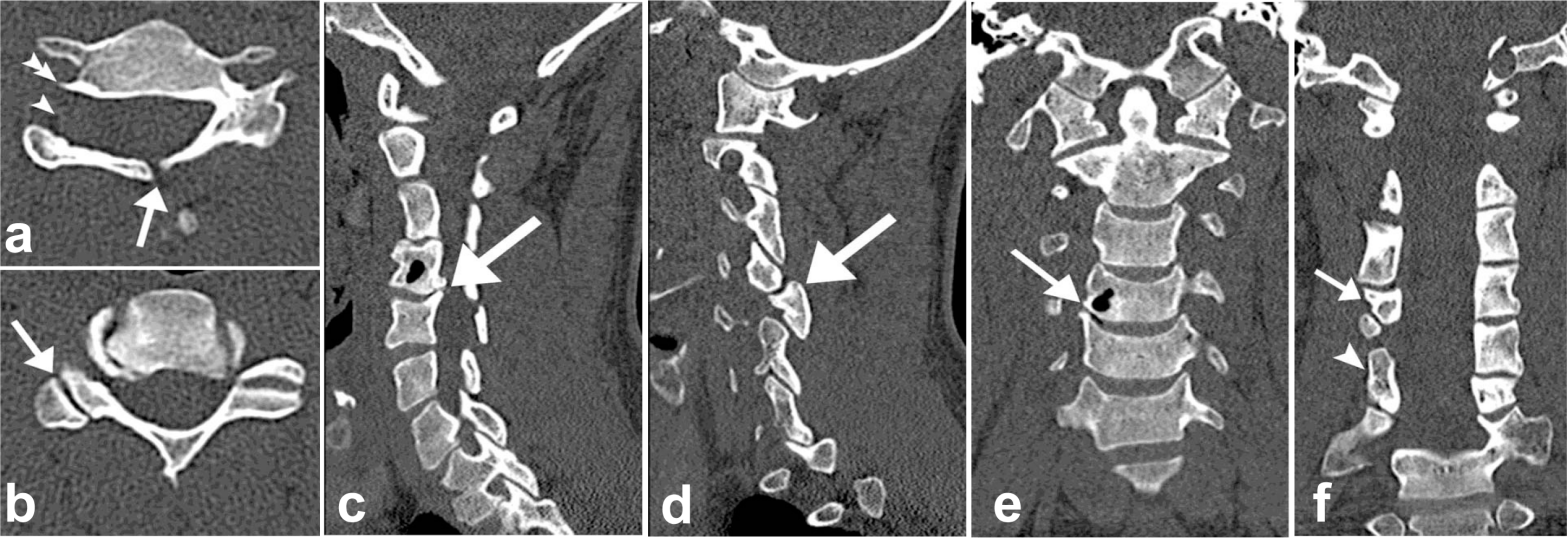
a-f: Computed Tomography of the cervical spine. Axial computed tomography (CT) images (a, b) and reconstructed images in the sagittal (c, d) and coronal (e, f) planes. Axial images at the level C5 show absent right cervical pedicle (arrowhead in a), spina bifida occulta (arrow in a) and dysplasia of the ipsilateral transverse process (double arrowhead in a) as well as reversed facet-joint (arrow in b). Images in the sagittal (c, d) and coronal (e, f) planes show degenerative changes at the level C4-5 (arrow in c) with formation of a bone cyst in C4 (arrow in e). The dorsal displacement of the articular pillar and reversal of the ipsilateral facet articulation (arrow in d) as well as hypoplasia of the pillar of the supra-adjacent (arrow in f) and hyperplasia of pillar of the infra-adjacent vertebra (arrowhead in f) are also well appreciated on the coronals.
